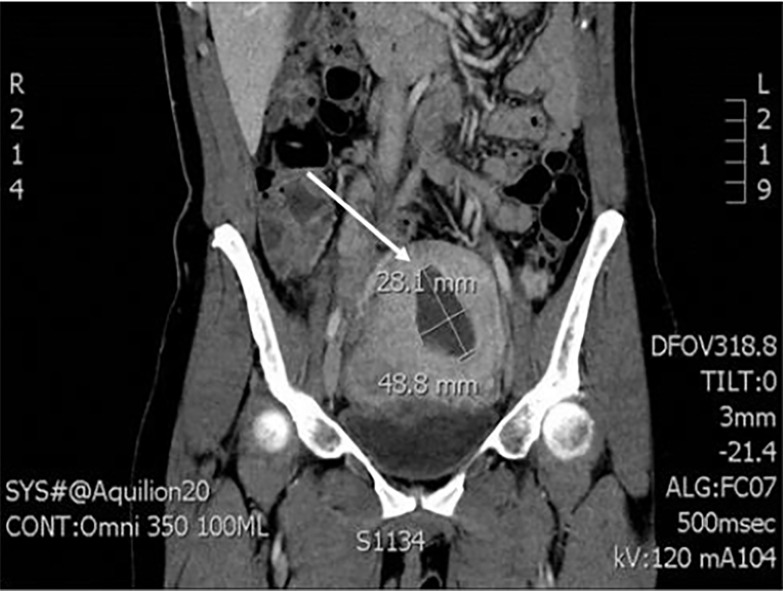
Contrasted computed tomography with the white arrow demonstrating the 2.8 cm × 4.8 cm intrauterine fluid collection.
